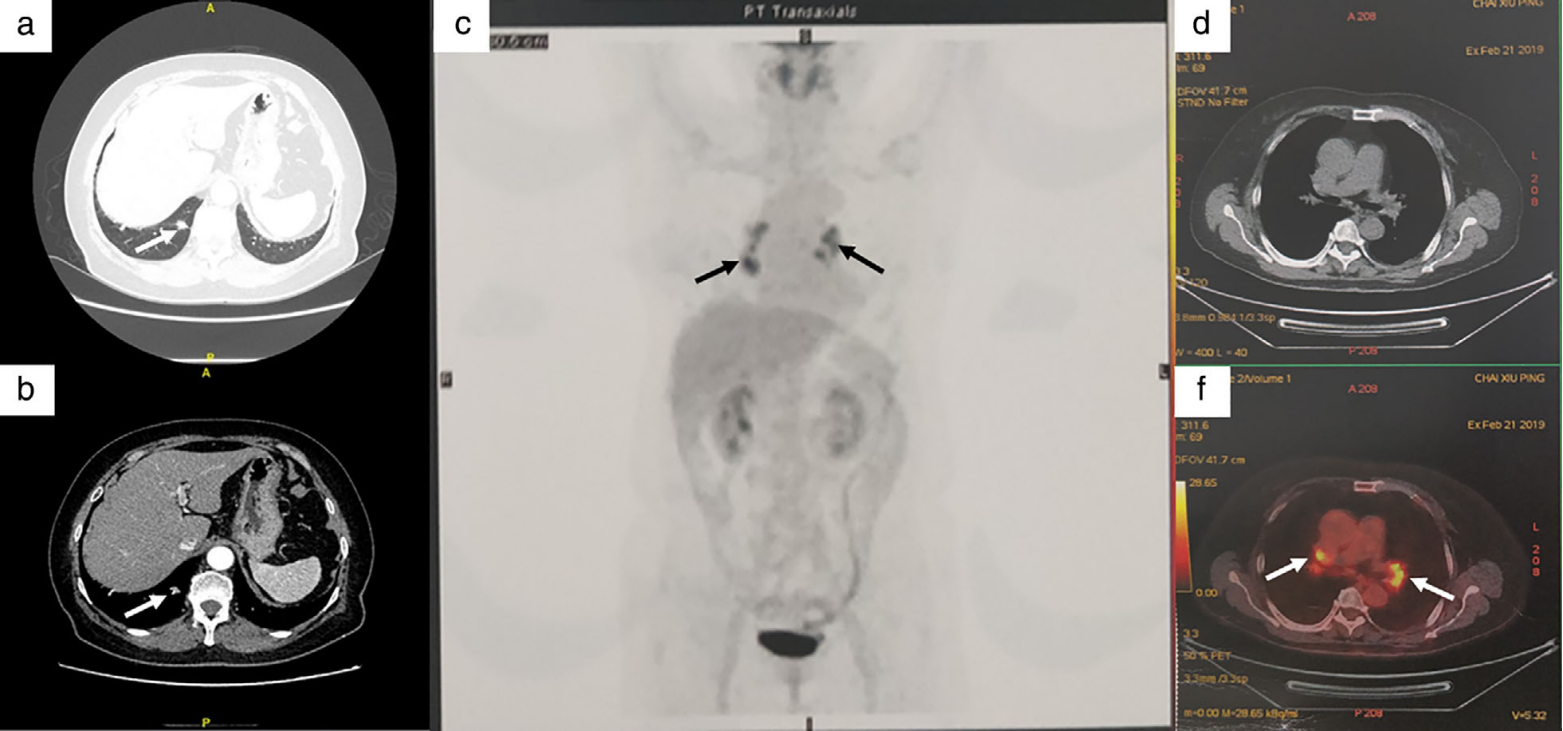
(a, b) Contrast‐enhanced computed tomography (CT) indicated an irregular solid nodule with a maximum diameter of 1.2 cm in the dorsal segment of the right lower pulmonary lobe. (c, d, e) 18F‐FDG CT‐PET showed focal hyperaccumulation within the pulmonary artery and hilum and mediastinal lymph nodes (LNs)
